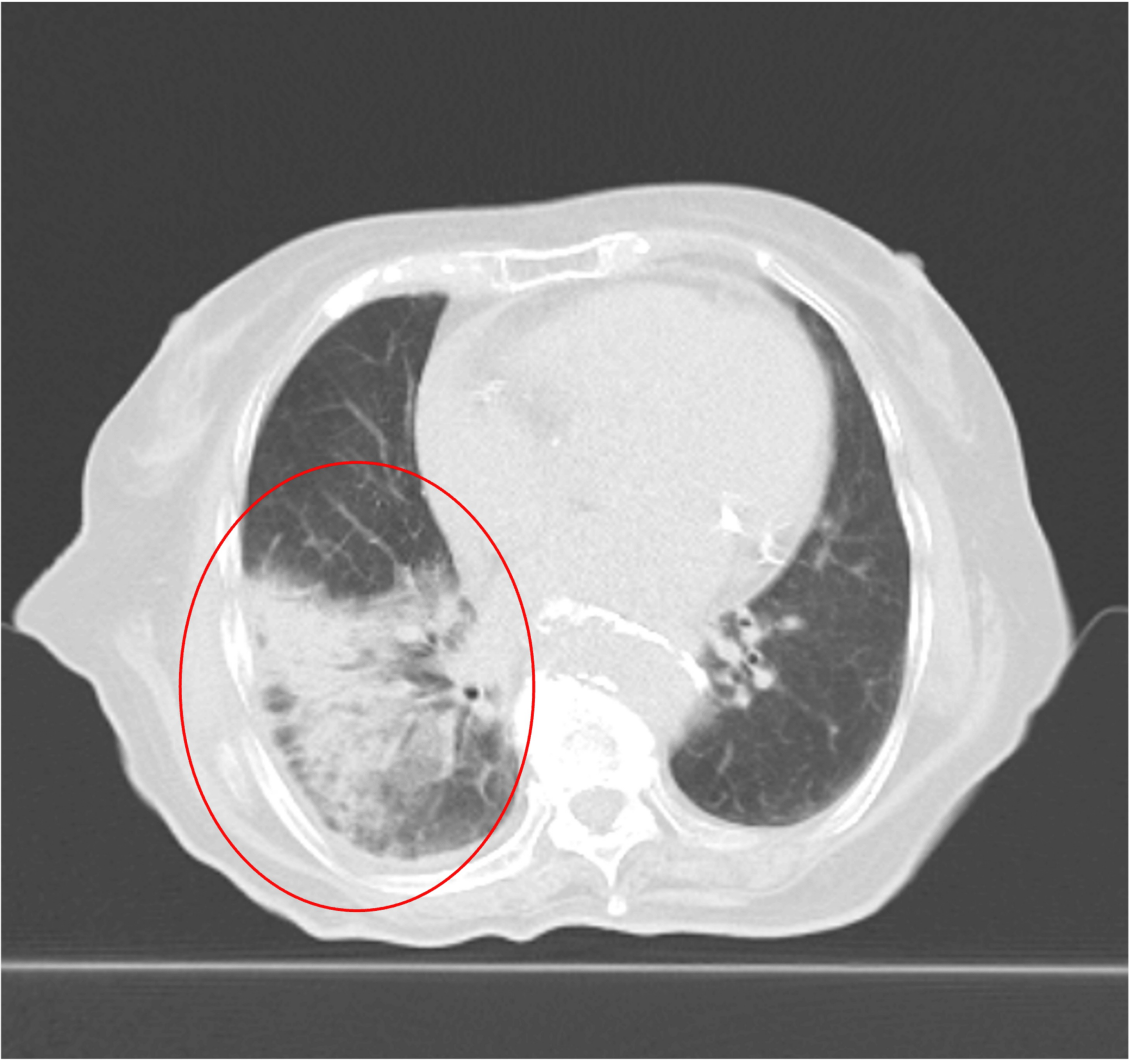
CT imaging four months after SBRT showing extensive pulmonary infiltrates CT 4 months after SBRT showed wide infiltration shadows inside and outside the irradiation range SBRT: stereotactic body radiotherapy; CT: computed tomography
